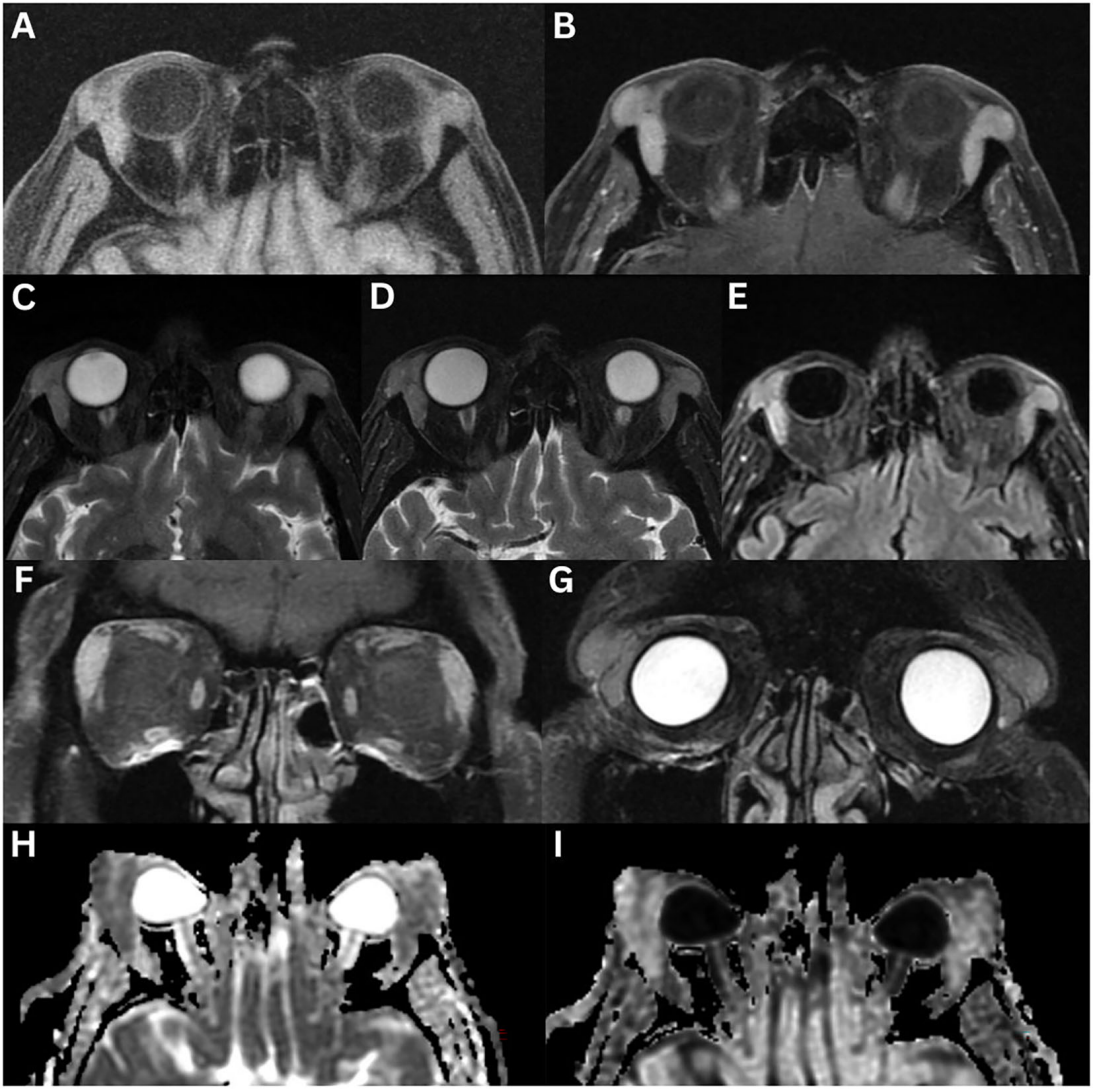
Sarcoidosis with bilateral lacrimal gland involvement. MRI T1-weighted axial fat-suppressed FLAIR (A), axial fat-suppressed contrast-enhanced (B), T2-weighted axial (C), axial fat-suppressed (D), axial fat-suppressed FLAIR (E), T1-weighted coronal fat-suppressed contrast-enhanced (F), and T2-weighted coronal fat-suppressed (G) sequences demonstrate asymmetric bilateral enlargement of the lacrimal glands, involving both orbital and palpebral lobes. The glands exhibit normal signal intensity on T1- and T2-weighted imaging, with normal homogeneous enhancement following contrast administration. Restricted diffusion is evident on axial ADC (H) and axial eADC (I).
